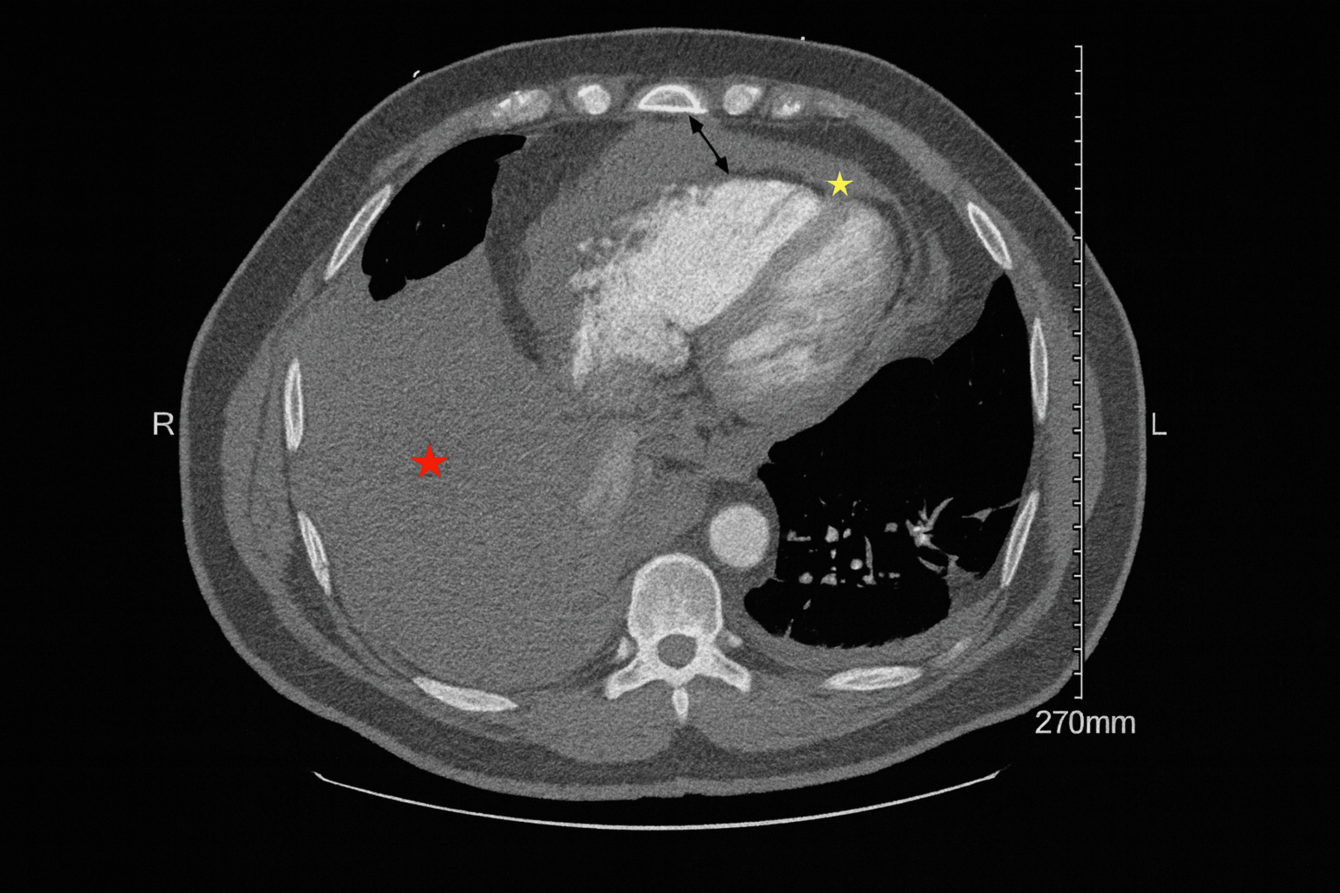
CT angiography of the chest: pericardial thickening (black arrow), moderate pericardial effusion (yellow star), and a large right pleural effusion (red star)
